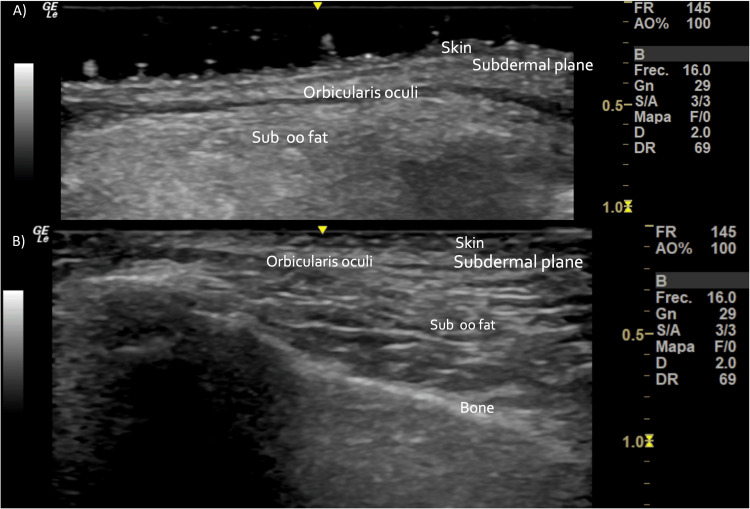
Skin and soft tissue high-resolution ultrasound before treatment (A), immediately after treatment (B). A slight increase in tissue echogenicity is visualized in all the tissue planes.
